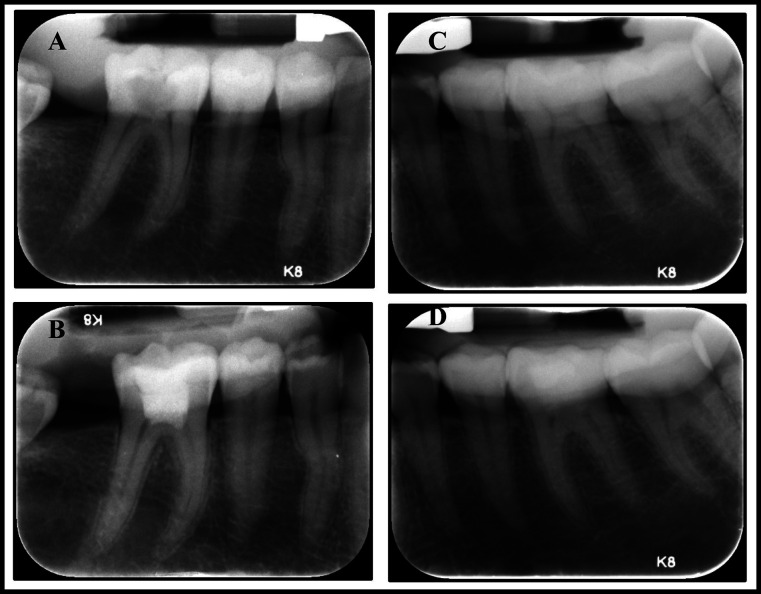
Preoperative and postoperative Radiographs of mandibular first molars treated with full pulpotomy A) Preoperative of case assigned for Portland cement pulpotomy, B) Postoperative after Portland cement full pulpotomy, C) Preoperative of case assigned for Biodentine pulpotomy, D) Postoperative after Biodentine full pulpotomy.
